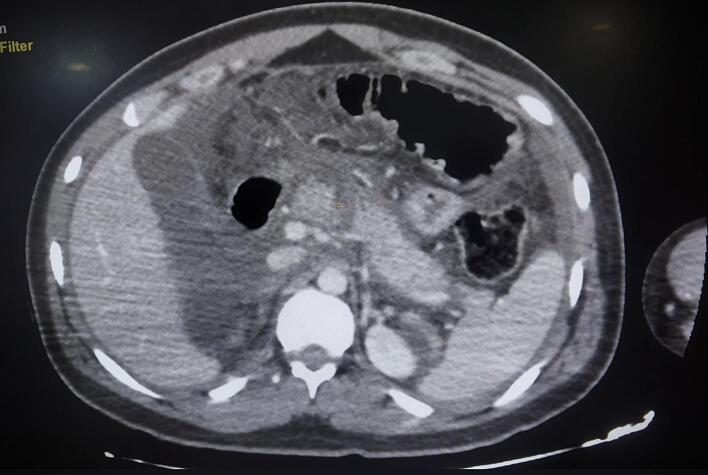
Abdominal CT scan showing complete transection of the isthmus of the pancreas (AAST 3).
